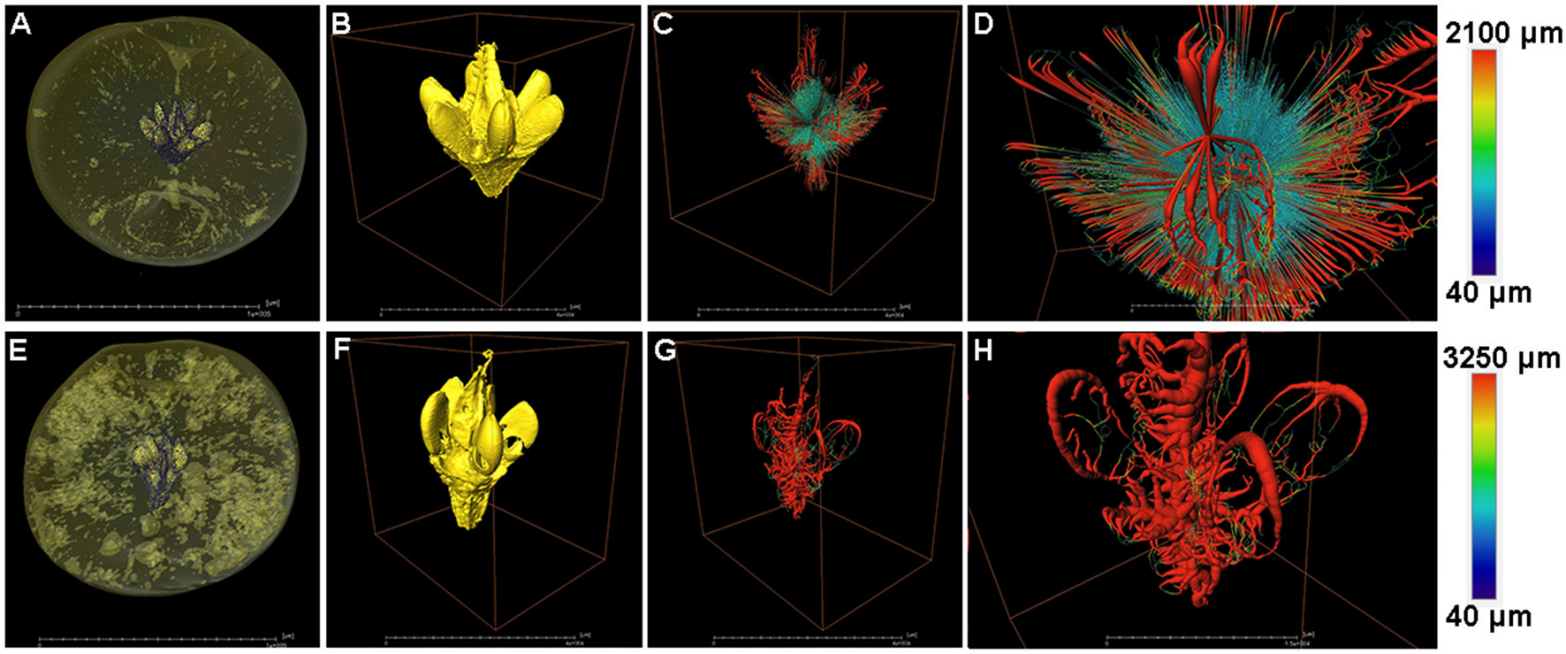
Structure and network skeleton analysis of “Akizuki” fruit core. (A,E) Renderings of X-ray scanned healthy and cork spotted fruit, respectively, where the fruit core was distinguished by the grayscale values. (B,F) Reconstructed 3D model of the healthy fruit core and cork spotted fruit core, respectively. (C,G) Reconstructed 3D network model of (B,F), respectively. (D,H) Closer view of (C,G), respectively. Color bars on the right indicate pore thickness from narrow (blue) to wide (red).
